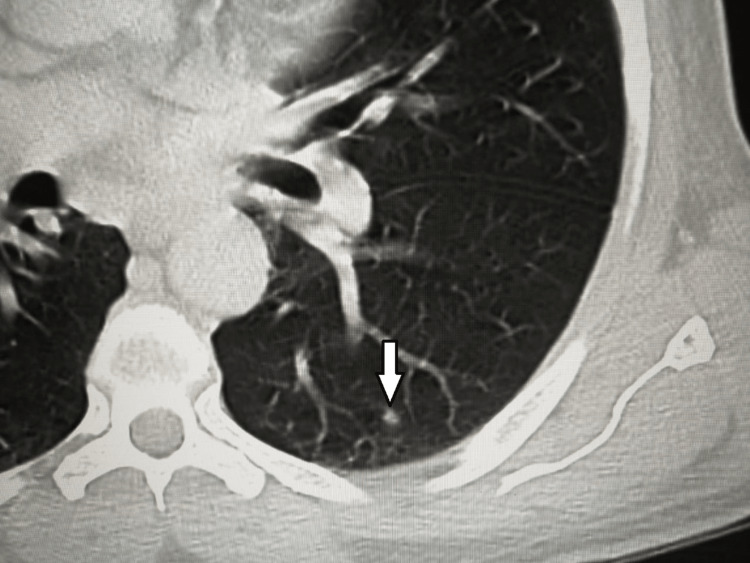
A CT scan of the chest The scan shows a small nodule-like consolidation in the lower lobe of the left lung (white arrow).
